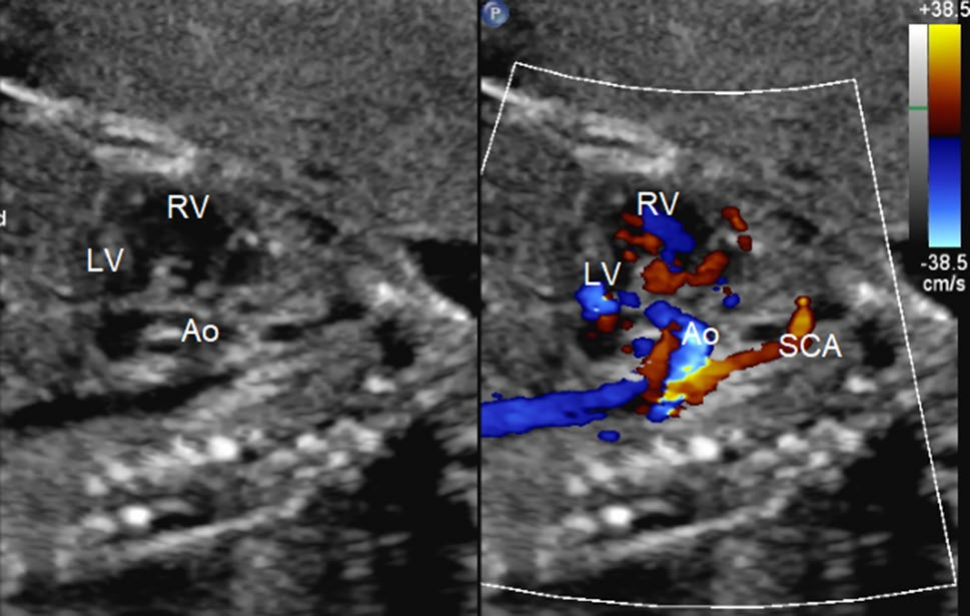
Fetal long axis echocardiographic view with 2D and color showing the superior–inferior relationship of the ventricles, the short ascending aorta, and an elongated subclavian artery. *LV* left ventricle, *RV* right ventricle, *Ao* aorta, *SCA* subclavian artery
